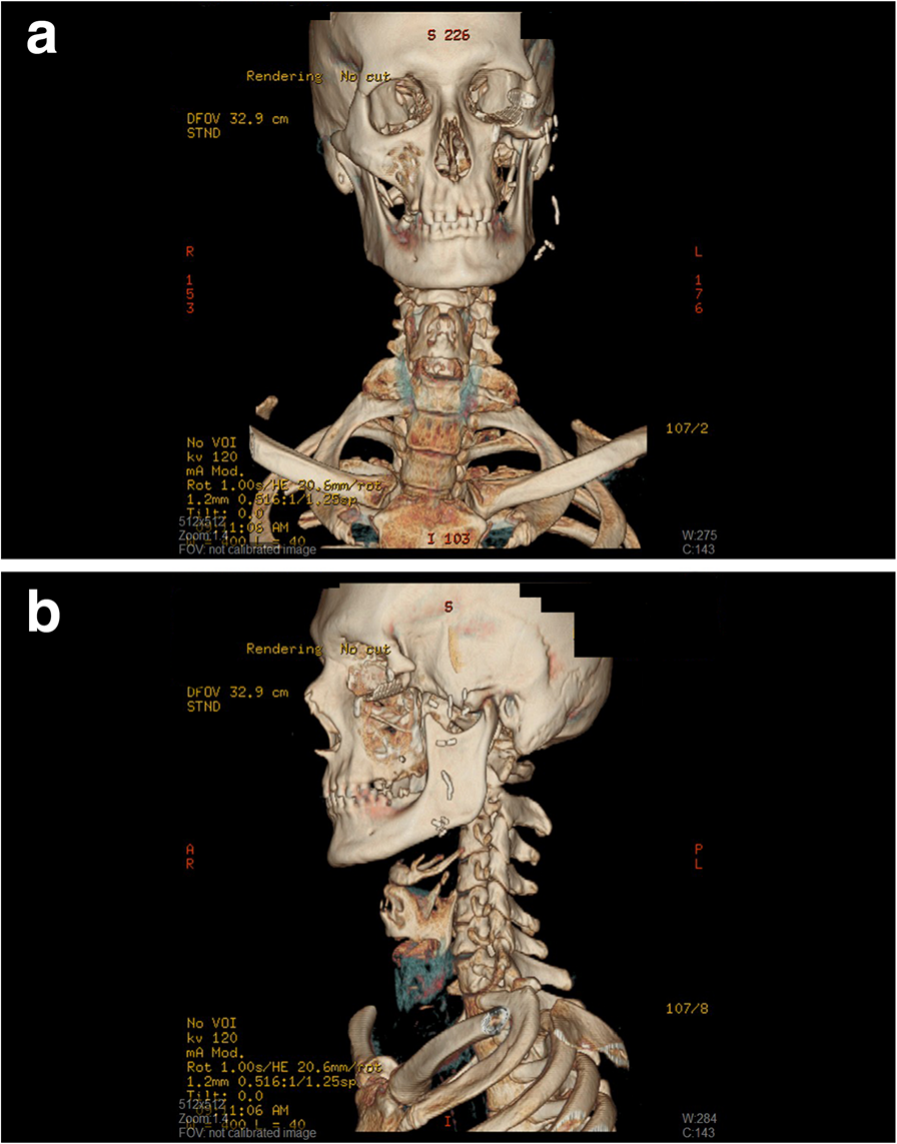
The tumor-free status revealed by CT 6 months after the operation in frontal (a) and lateral (b) view
